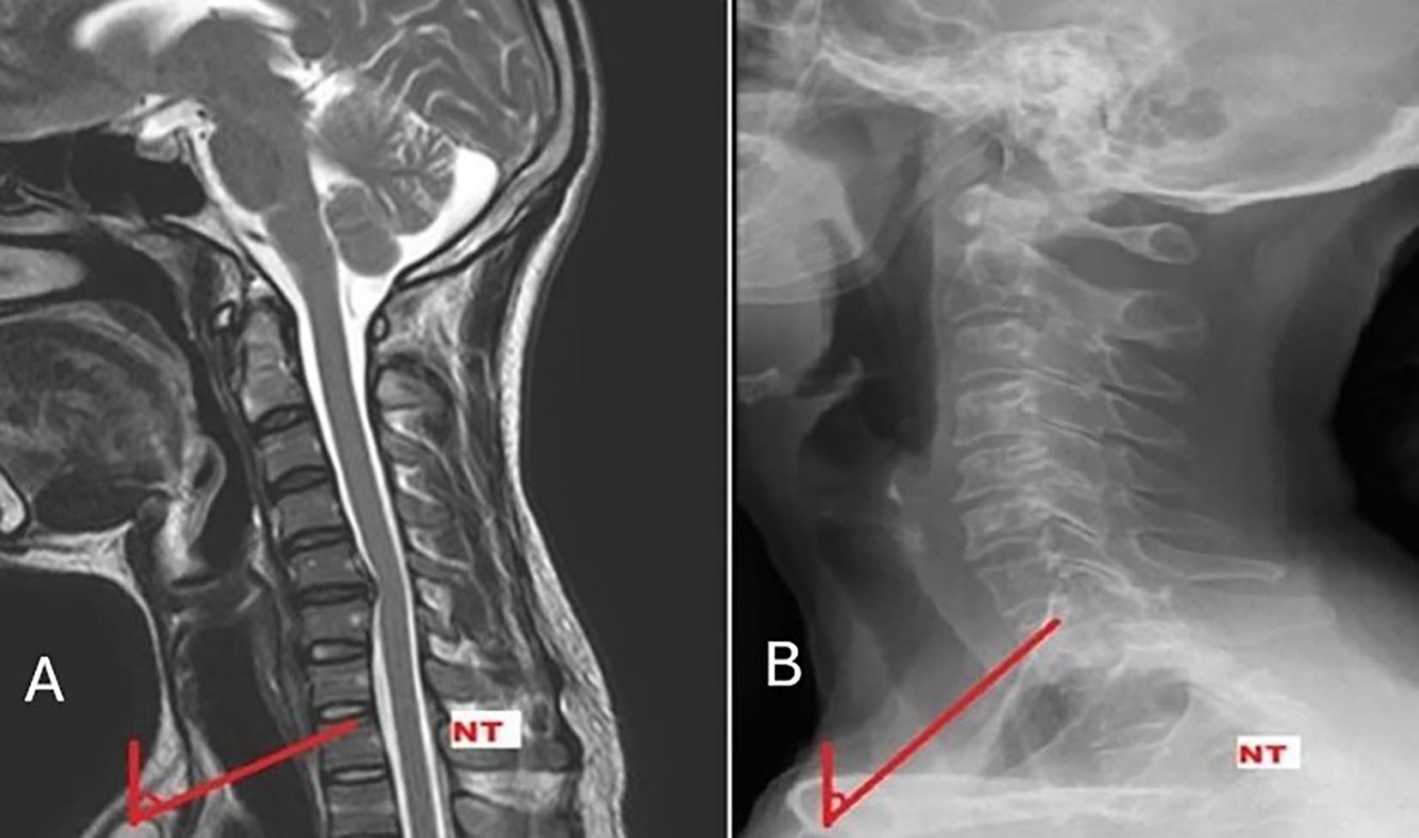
Measurement of neck tilt on MRI (A), and radiograph (B).
